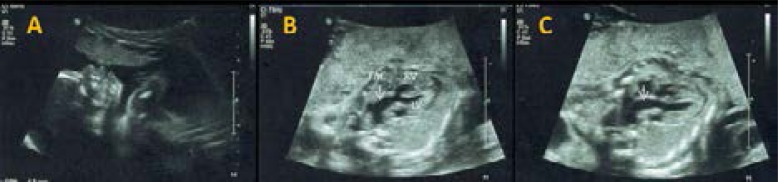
TAC ultrasound imaging. A) Fetal aspect (F1). B and C)Truncus arteriosus. Persistent common arterial trunk with agenesis of the pulmonary artery trunk and IVC.
